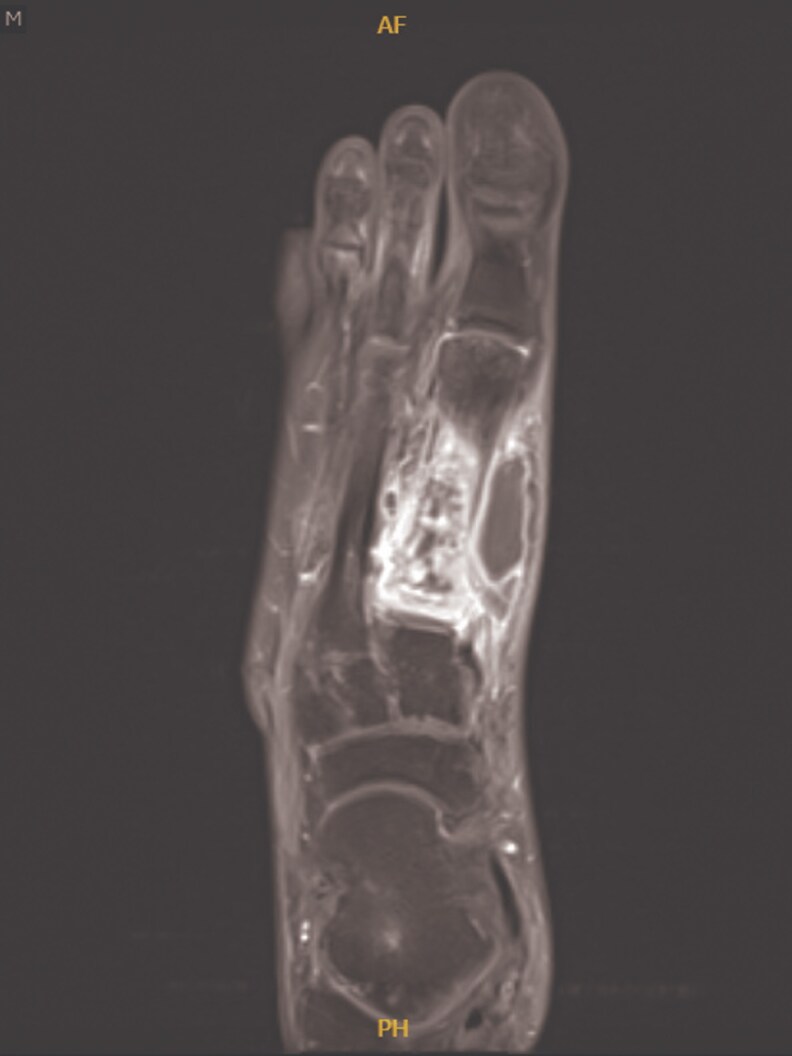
Imaging (eg, MRI or X-ray) depicting a metatarsal lesion associated with osteomyelitis in a *M riyadhense* infection (case 7; Table 2).
